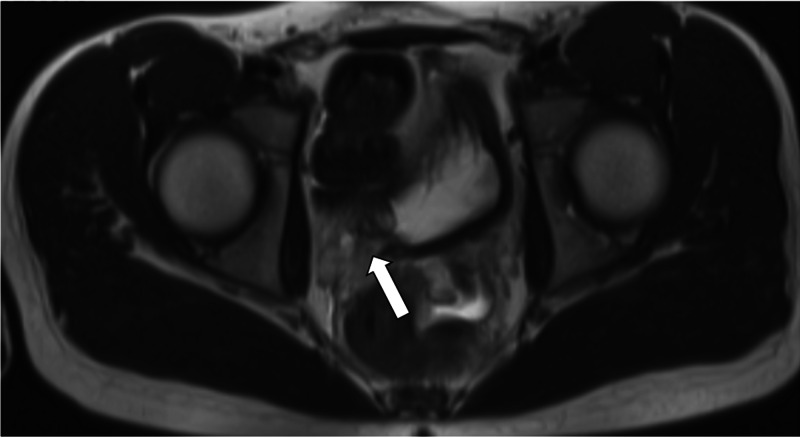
Axial view of HASTE pelvic MRI demonstrating approximately three millimeter ureteral stone near the right ureterovesicular junction with mild ureteral dilatation (white arrow). HASTE, Half-Fourier acquisition single-shot turbo spin echo
